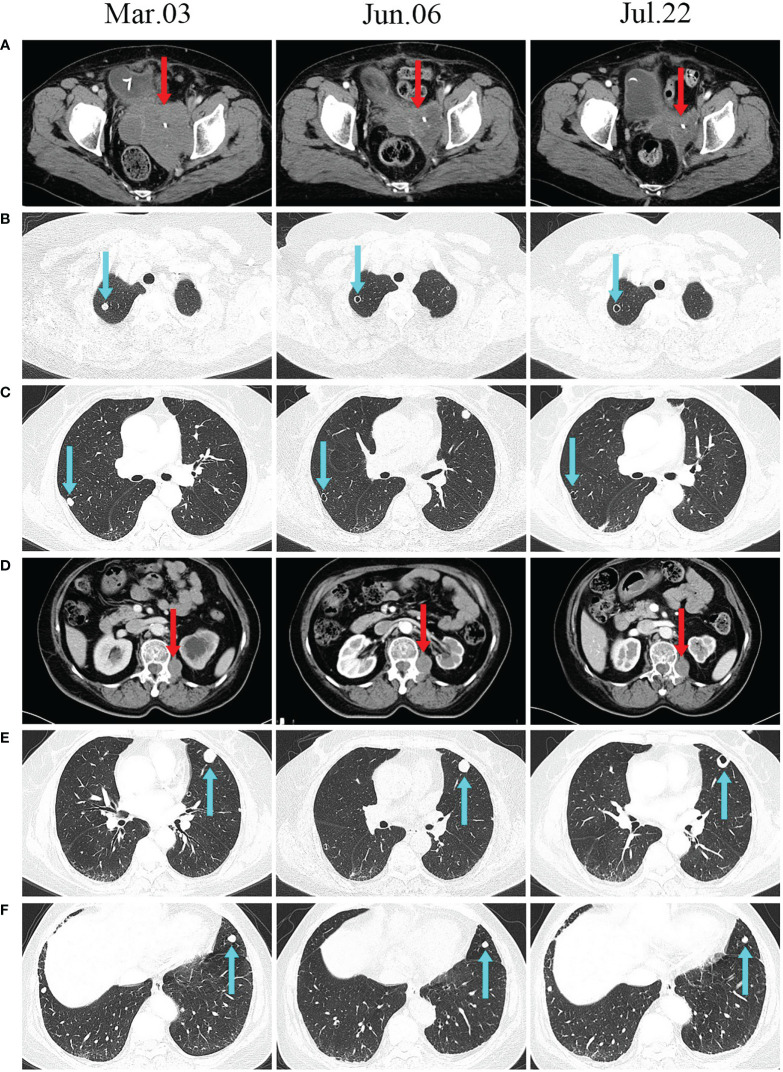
CT scans before and after treatment. (A, D) CT scans show changes in the size of irradiated lesions. (B, C, E) Unirradiated lung lesions developed cavities after treatment. (F) The unirradiated lesions in the lungs gradually decreased after treatment.
